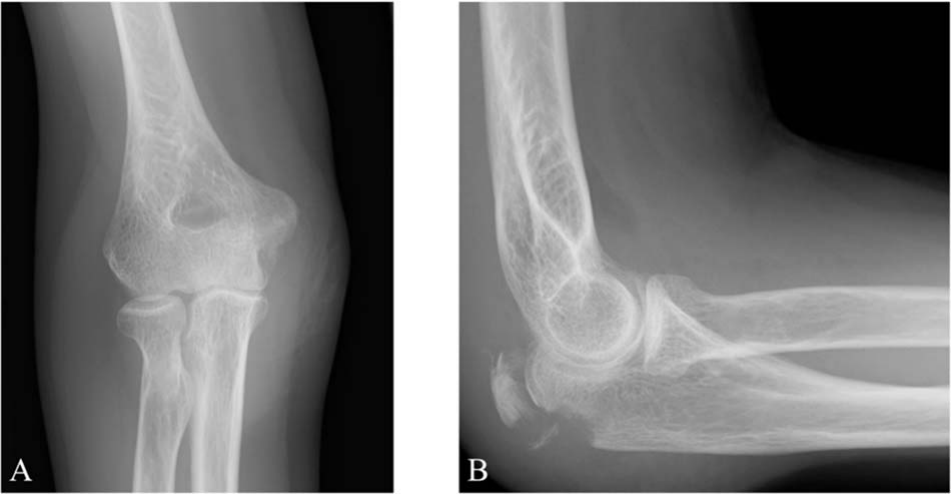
Plain radiographs of the right elbow show no abnormal findings in the anteroposterior view (A); however, an avulsion fracture of the olecranon is observed in the lateral view (B).
